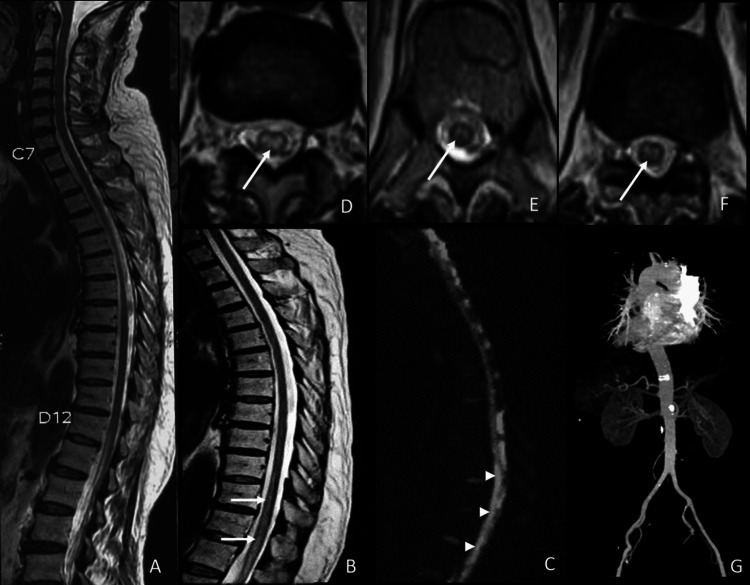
MRI of the spinal cord Normal T2-weighted sagittal MR image (A) at three hours. At five hours, sagittal T2-weighted (B) and diffusion-weighted (C) MR images showed linear pencil-like hyperintensity in the anterior two-thirds of the chord from D10 to L1 level (white-arrow). Axial T2-weighted MR images (D-F) showing hyperintensity in the corresponding regions (arrowheads). CT angiography (G) shows few calcifications. MRI: magnetic resonance imaging
